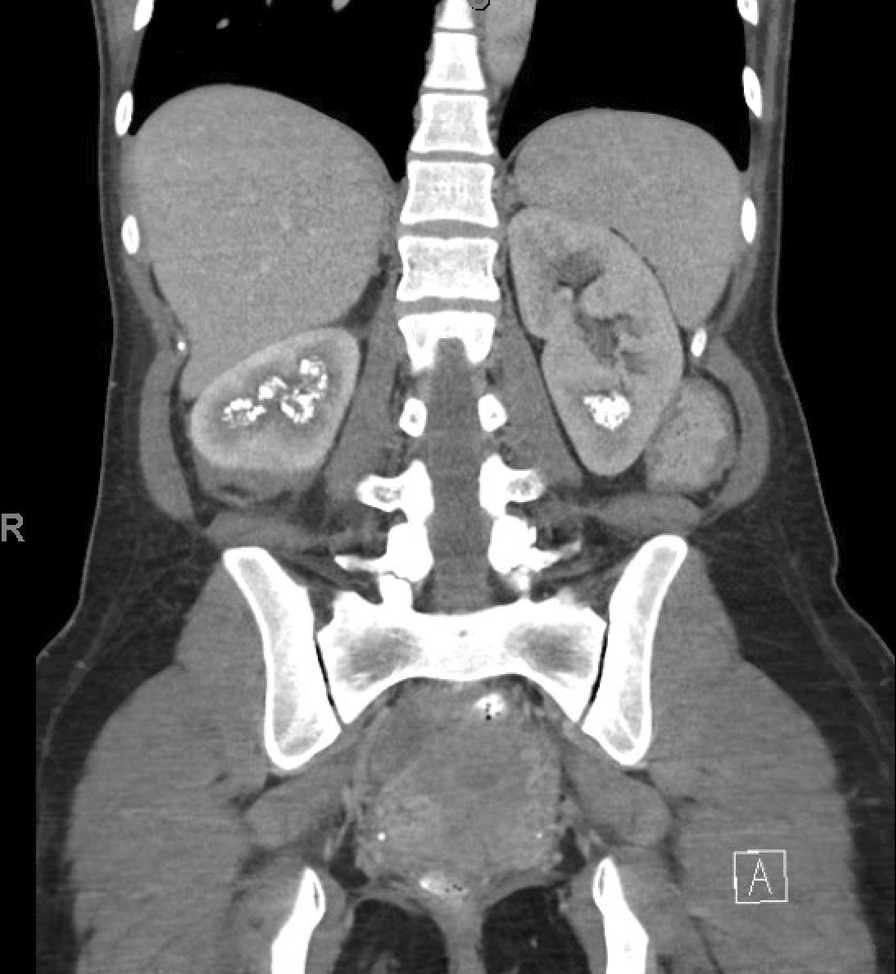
Coronal CT image demonstrating patient’s focal nephrocalcinosis prior to treatment
